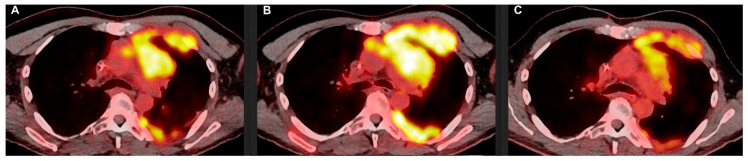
The left thoracic lesions with mediastinal, pericardial, pleural, and rib involvement in the baseline ^18^F-FDG PET/CT scan (A), as well as their evolution in the ^18^F-FDG PET/CT scan after the first 4 cycles of immunotherapy (B) and in the subsequent ^18^F-FDG PET/CT scan (C). SUXmax mediastinal mass and pleural lesion: A: 12.3 and 9.5; B: 13.2 and 13.7; C: 9.7 and 10.9.
